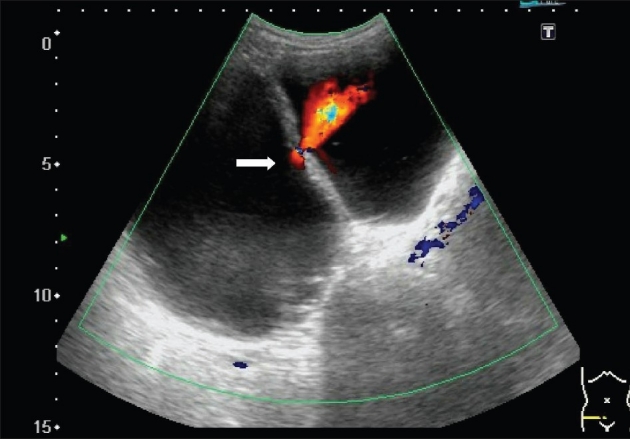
Sonography of the pelvis showing a large cystic lesion 9.5 cm in diameter (right) and a 0.25 cm small hole connecting the diverticulum (right) and the bladder (left) with a left-to-right jet phenomenon.
